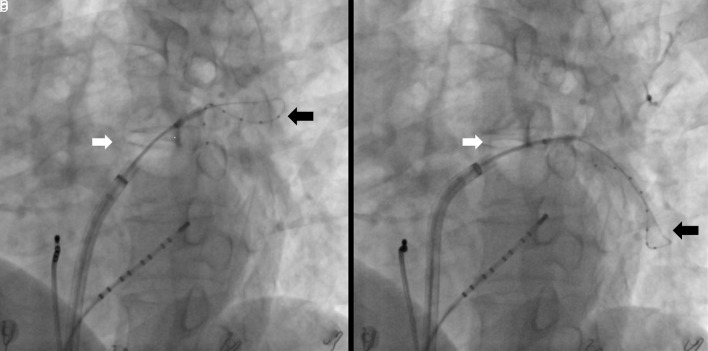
X-ray image of the relative position between the Achieve electrode (black arrow) and the cryoballoon (white arrow) during the cryoablation process. (a) The cryoballoon and Achieve electrode are both located near the pulmonary vein vestibule. (b) The cryoballoon is placed near the pulmonary vein vestibule, and the Achieve electrode penetrates deep into the pulmonary vein.
